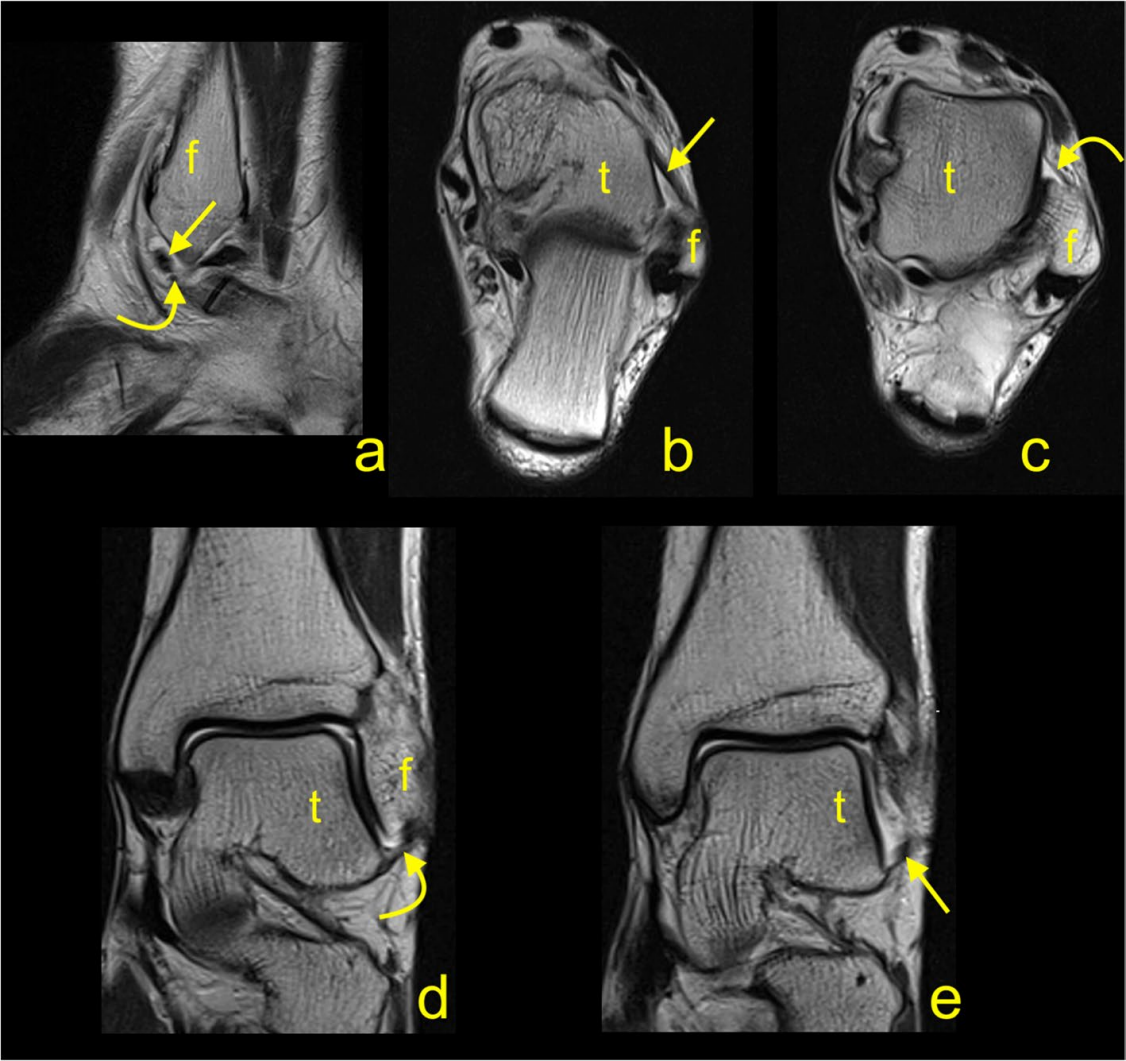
A double fascicular anterior talofibular ligament. Two symmetrical fascicles are visible: a superior fascicle (arrow) and an inferior fascicle (curved arrow). The dashed arrow shows the midportion of the CFL. A T2-weighted axial section; b T2-weighted coronal section; c T2-weighted sagittal section. F, fibula; t, talus
